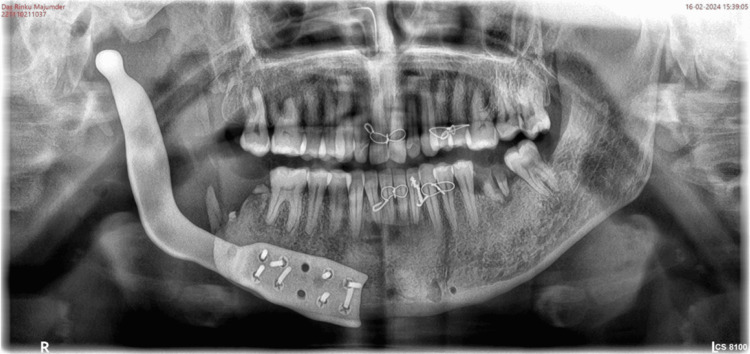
Post-operative OPG showing the successful placement of the PSI OPG - Orthopantomogram, PSI - Patient-specific implant
